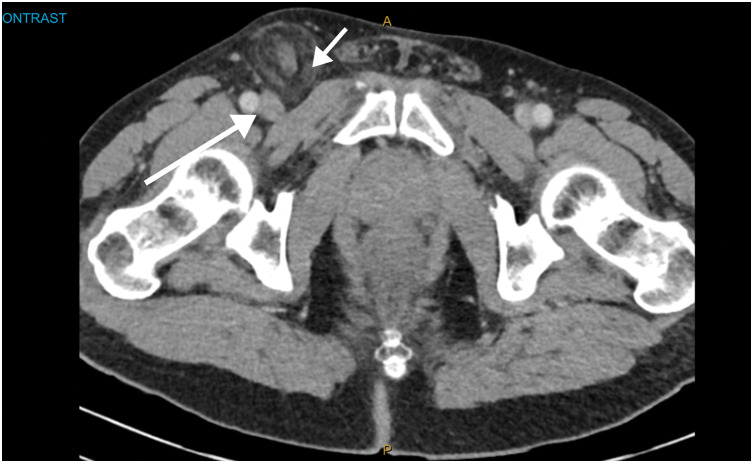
Axial CT confirming the appendix within the right femoral canal. The long arrow indicates the femoral vein, with the femoral artery lateral to it. The short arrow indicates the femoral hernia located below the inguinal ligament.
